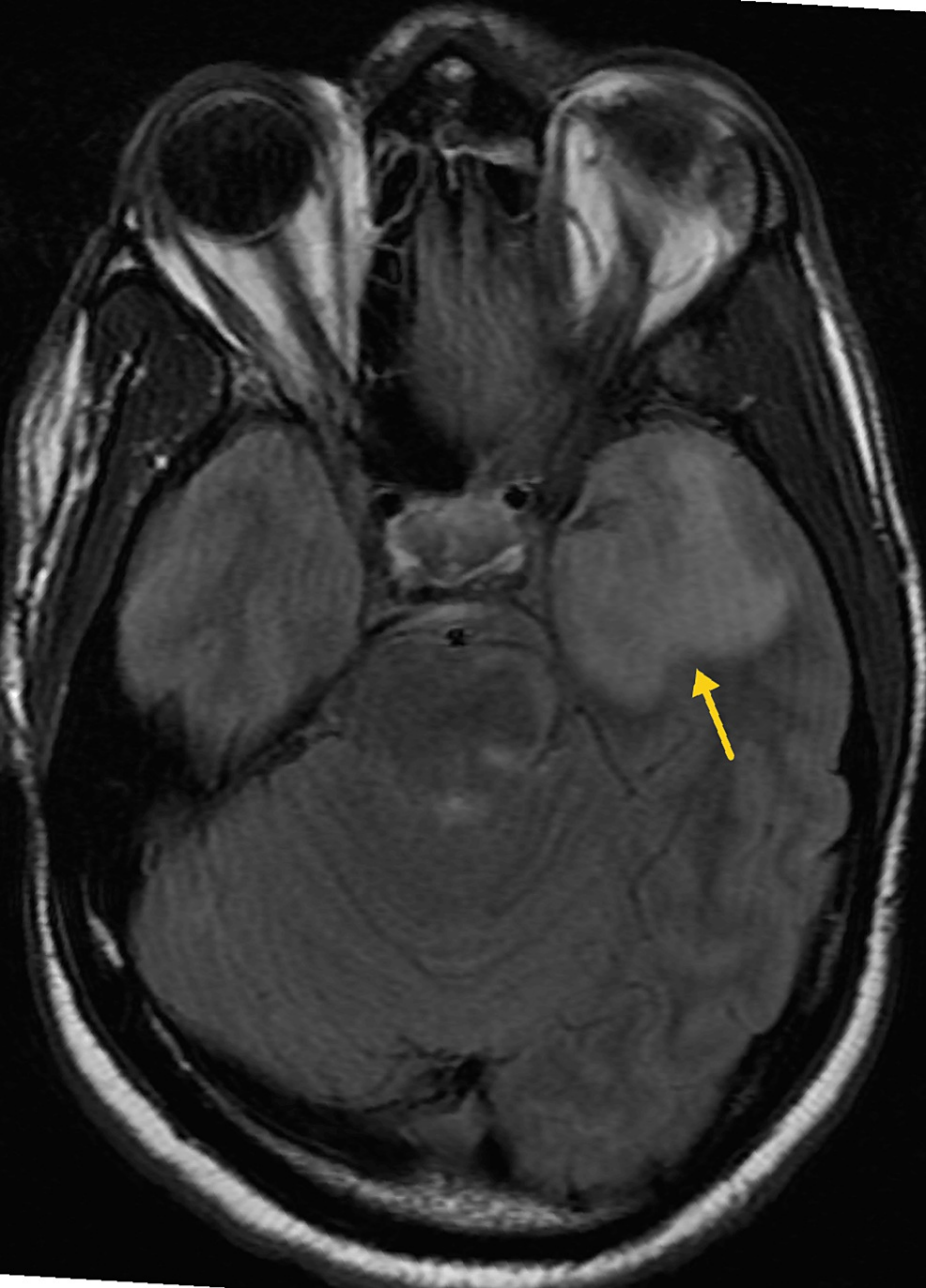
Representative axial fluid-attenuated inversion recovery (FLAIR) image through the level of the temporal lobes demonstrates extensive anterior left temporal edema consistent with cerebritis. Mild edema is also noted in the right temporal lobe.
